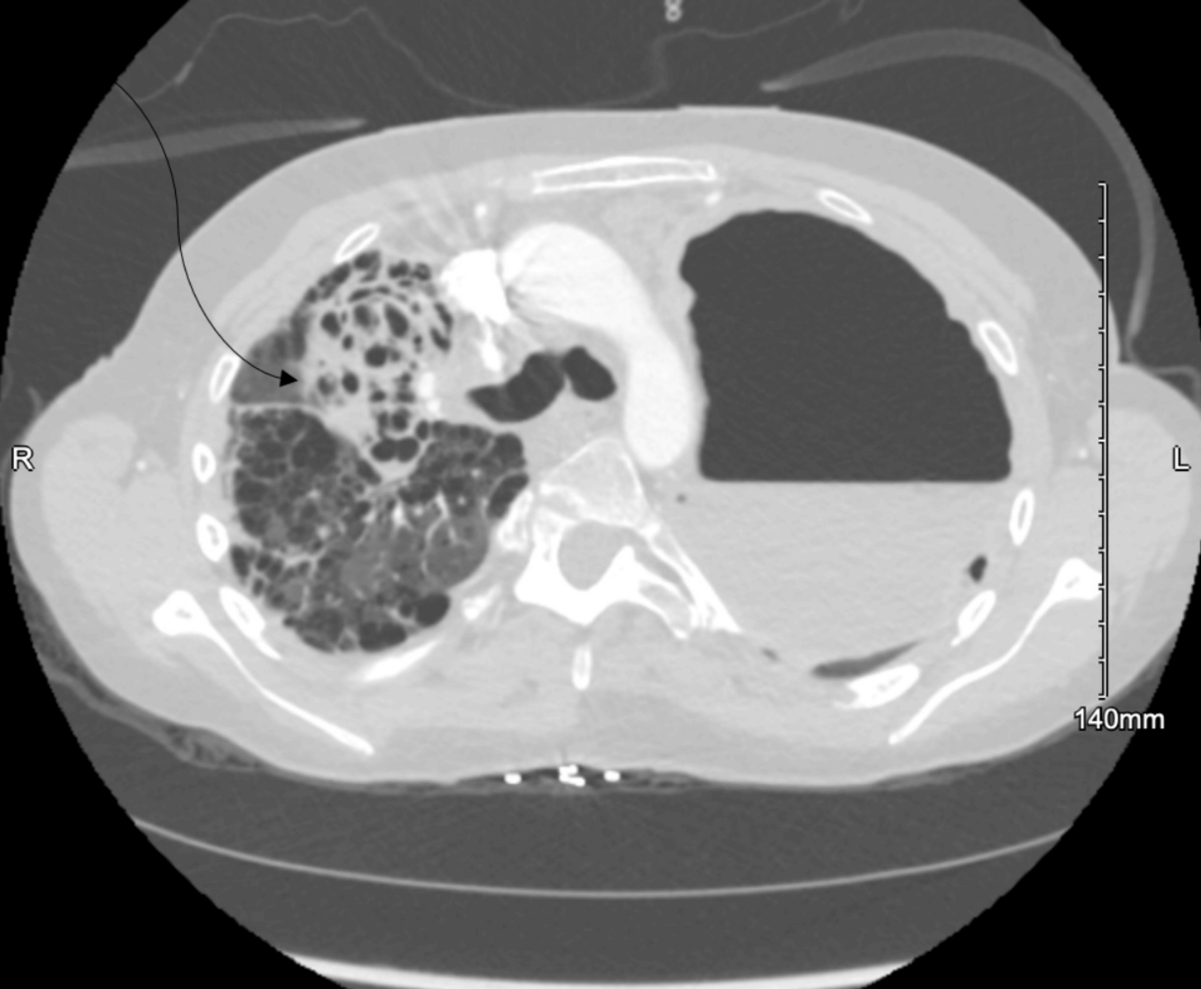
Computed Tomography Scan Demonstrating a Cavitary Lesion of the Right Upper Lobe and Apex The image shows a de-identified right upper lobe cavitary lesion in a patient with *STAT3*-deficient hyperimmunoglobulin E syndrome and secondary hypogammaglobulinemia. No identifying patient information is present, and informed consent for publication was obtained.
